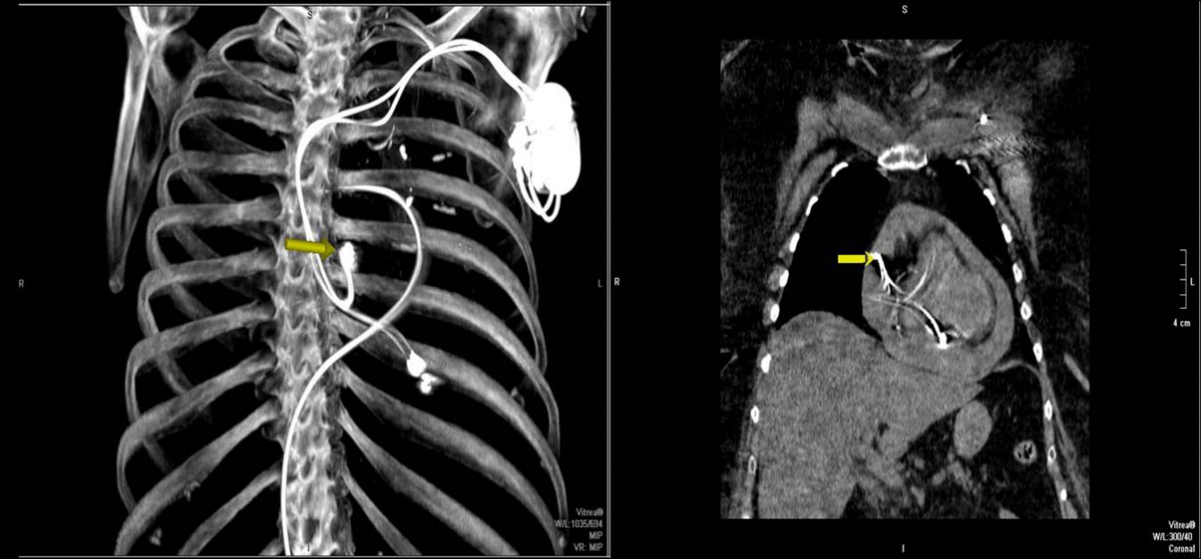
Multiplanar reformatted images from unenhanced CT of the chest demonstrate dense pericardial fluid consistent with hemopericardium. The atrial lead (yellow arrow) is noted to extend into the pericardial fluid (upper right image) with imaging limited due to motion artifact and streak artifact. Note: The right ventricular lead can also be seen on this coronal image which appears to be within the wall of the right ventricle. Maximum intensity projection image (lower left image) demonstrates the presence of a dual-lead pacemaker as well as a femoral-route pulmonary artery catheter.
